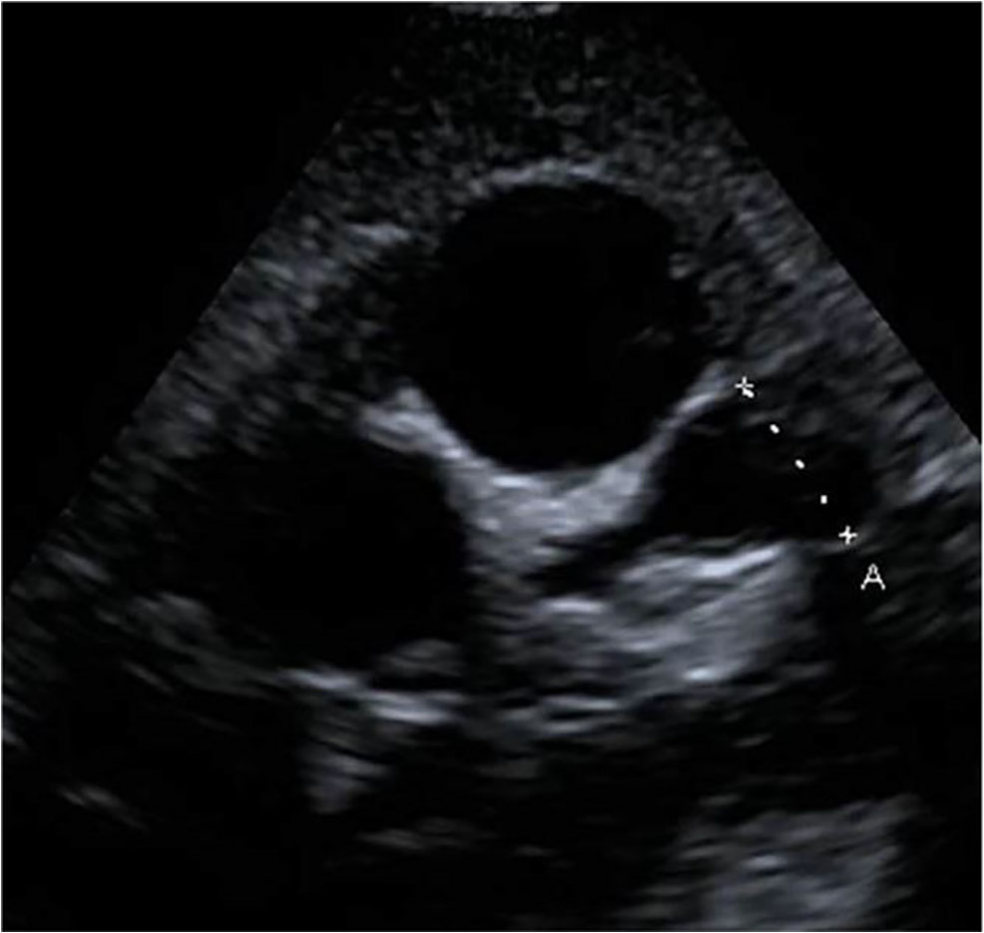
Transthoracic echocardiography, parasternal short-axis view. Giant aneurysm (A = 10 mm) of the LAD in a 3-year-old girl with Kawasaki disease
